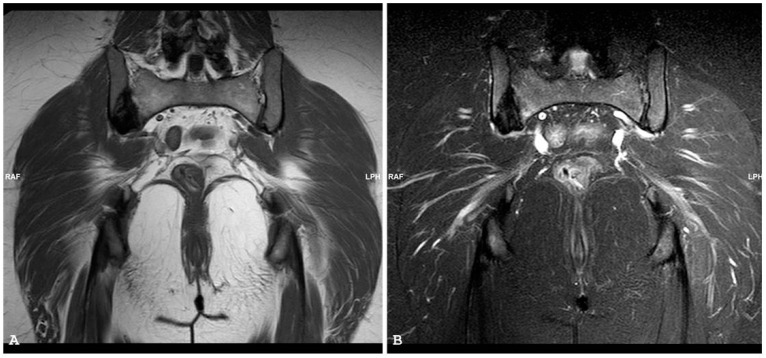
Coronal T1-weighted (A) and coronal short tau inversion recovery (B) magnetic resonance imaging sequences show the triangular areas of sclerosis along the inferior iliac margins of the right sacroiliac joint.
